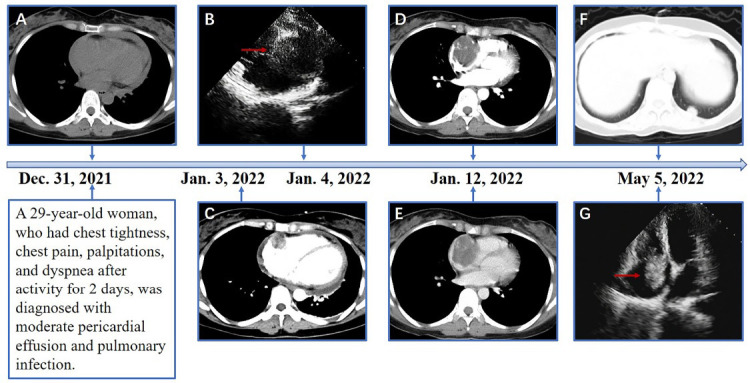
The timelines of this radiological examination. (A) The chest CT scan showed pericardial fluid and no obvious abnormalities concerning the heart. (C) Accidentally, an incidental abdominal CT with an enhancement scan suggested a filling defect in the right atrium. (B) The subsequent echocardiography revealed an isoechoic mass measuring 54mm × 42mm in the right atrium and right auricular region. (D, E) Arterial phase imaging and venous phase imaging revealed a marked inhomogeneous progressive enhancement of the right atrial mass with vascular shadows. (F) A lung window image showed a metastatic nodule in the lower left lung. (G) Echocardiography results of the reexamination revealed an isoechoic mass.
